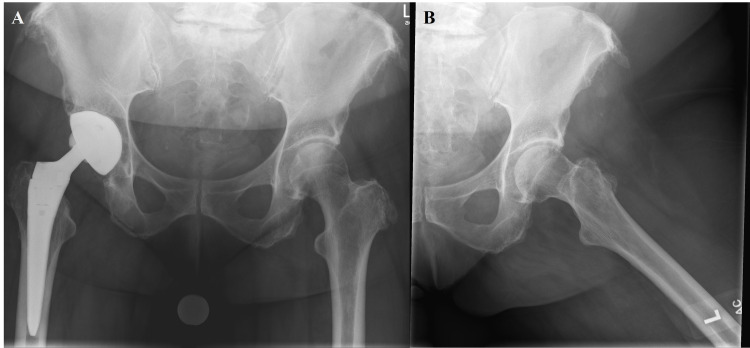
Preoperative anterior-posterior (A) and lateral (B) hip radiographs demonstrating moderate left hip osteoarthritis and a right total hip arthroplasty with a modular neck construct.
